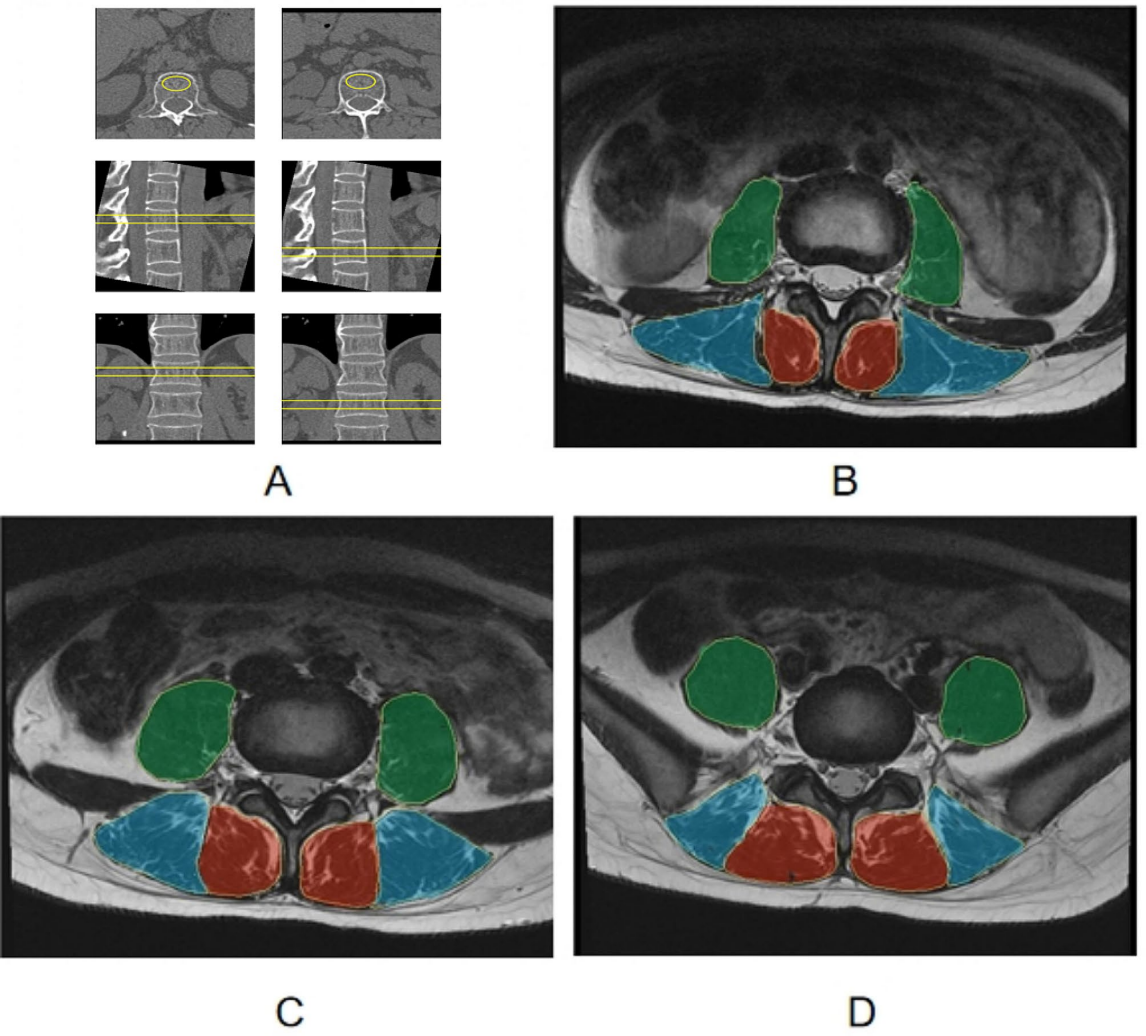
ROI delineation for measuring the vBMD and paraspinal muscle PDFF and CSA. ROIs for vBMD measurements in L1 and L2 vertebral bodies were drawn automatically on the QCT pro workstation in three-plane images with manual adjustment to avoid the cortical bones (A). T2-weighted images were utilized to delineate ROIs for measuring the CSA and PDFF value. ROIs for the bilateral paraspinal muscles (MF in red, ES in blue, and PS in green) were manually delineated at the central level of L3/4 (B), L4/5 (C), and L5/S1 (D) on axial T2-weighted images. Subsequently, the same ROIs were automatically copied to the IDEAL-IQ images and then simply adjusted to measure PDFF values. vBMD, volumetric bone mineral density; PDFF, the proton density fat fraction; CSA, cross-sectional area; QCT, quantitative computed tomography; ROI, region of interest; MF, multifidus; ES, erector spinae; PS, psoas major
